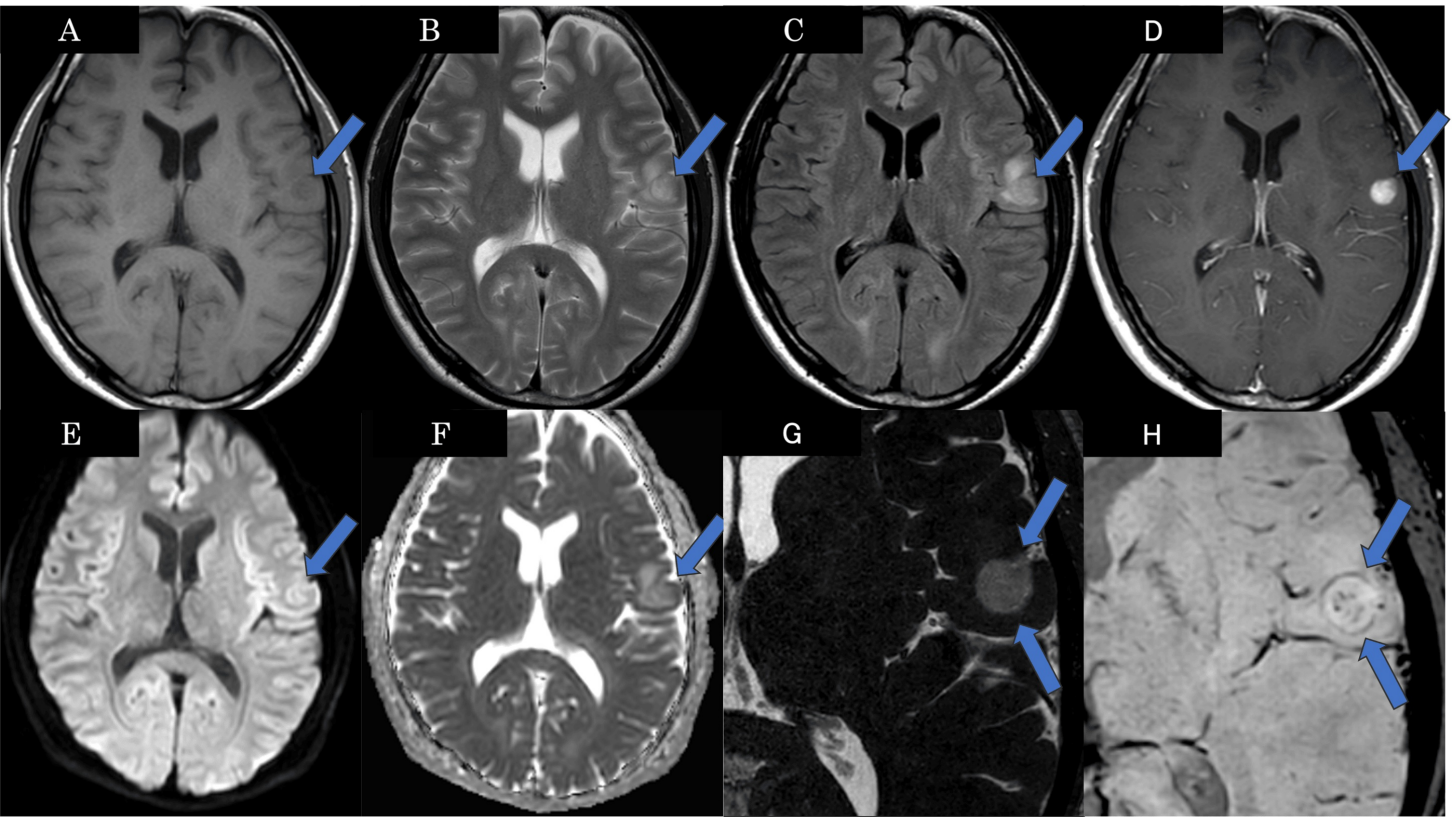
Brain MRI of the patient The well-circumscribed mass (arrows) appears hypointense on T1WI (A), hyperintense on T2WI (B) and FLAIR (C), and demonstrates solid enhancement on contrast-enhanced T1WI (D). There is no signal elevation on DWI (E), and the apparent diffusion coefficient (ADC) map (F) shows a mean ADC value of 1.10×10-3 mm²/sec. Constructive interference in steady state (CISS) imaging (G) reveals no cystic component within the tumor. The SWI (H) demonstrated rim-like low signal intensity at the periphery and punctate low signal areas internally, indicative of hemosiderin deposition. T1WI: T1-weighted image, T2WI: T2-weighted image, FLAIR: Fluid-attenuated inversion recovery, DWI: Diffusion-weighted image, SWI: Susceptibility-weighted imaging
